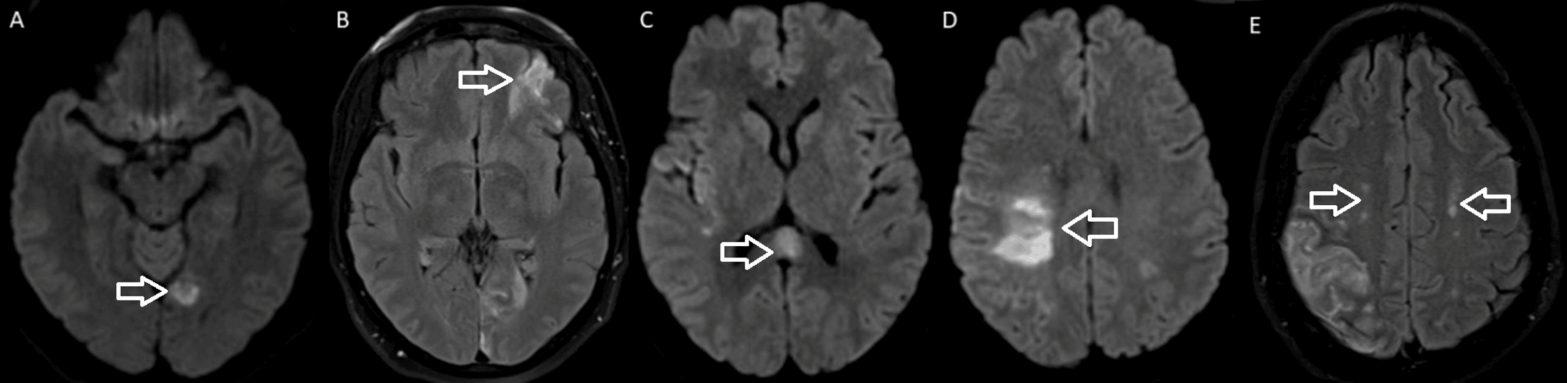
(A) DWI hyperintensity on the left medial occipital (arrow), (B) FLAIR hyperintensity on the left inferior frontal (arrow), (C) DWI hyperintensity on the corpus callosum (arrow), (D) DWI hyperintensity on the right posterior frontal and parietal (arrow), and (E) FLAIR hyperintensity on the bilateral anterior and inferior watershed areas (arrows) DWI: diffusion-weighted imaging, FLAIR: fluid-attenuated inversion recovery
